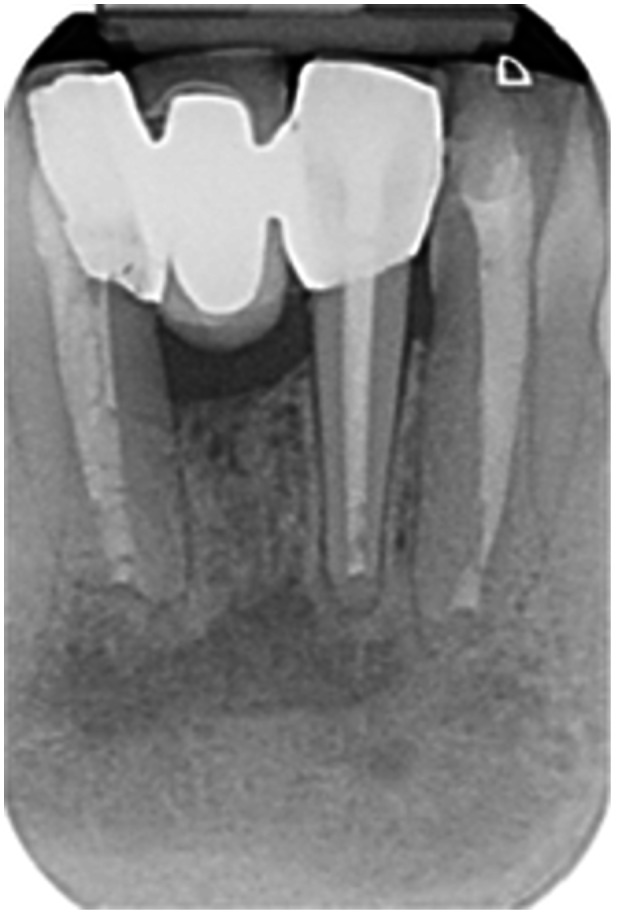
Case 12: The preoperative CBCT and periapical radiograph show an extensive periapical radiolucency associated with teeth 32, 31, 41, and 42. Tooth 41 was eventually extracted due to vertical root fracture, nonsurgical root canal treatment performed on teeth 42 and 31, and nonsurgical root canal retreatment on tooth 32. Due to persistent infection, apical surgery was performed on teeth 42, 31, and 32. At the 6‐month follow‐up, a significant reduction in the size of the periapical radiolucency can be seen, with PDL reattachment noted at teeth 42, 31, and 32 and complete healing of the apical tissues of tooth 32.
